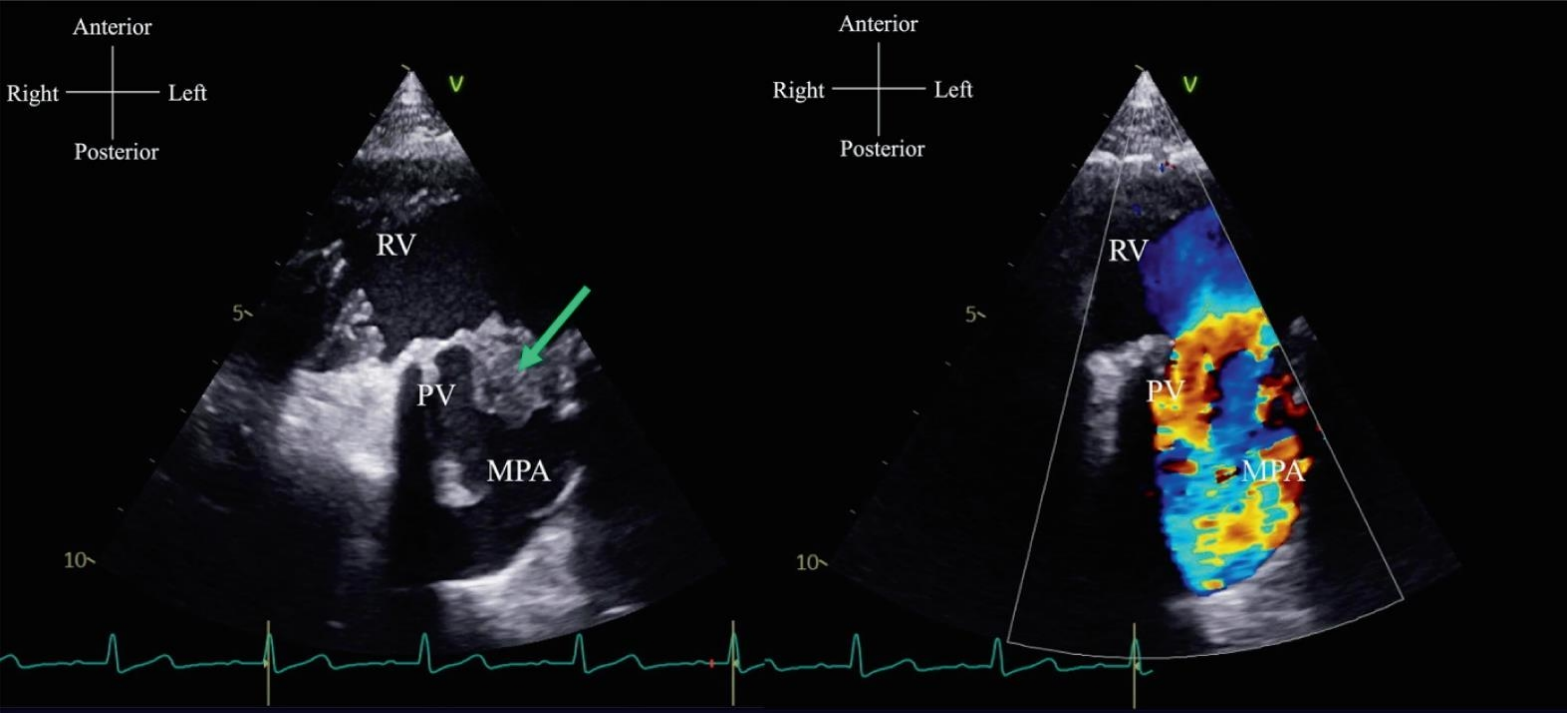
TTE in parasternal short axis view showing a large vegetation (arrow) on the bioprosthetic PV in 2D (left) and mild PV stenosis in color (right). (RV = right ventricle, PV = pulmonary valve, MPA = main pulmonary artery).
